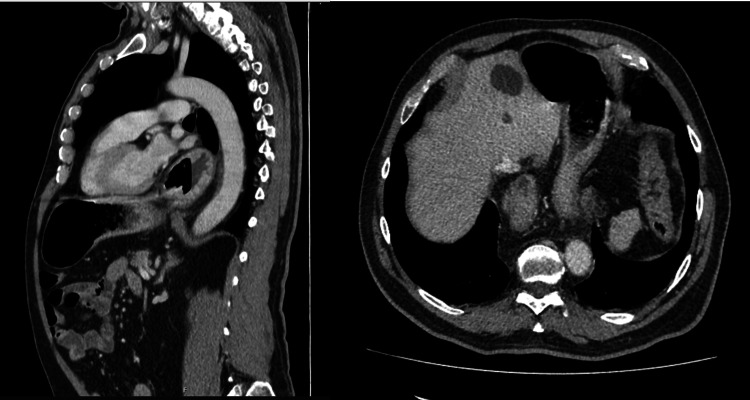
Computed tomography (sagittal and transverse section) performed during the follow-up period A partial recurrence of HH was documented.
